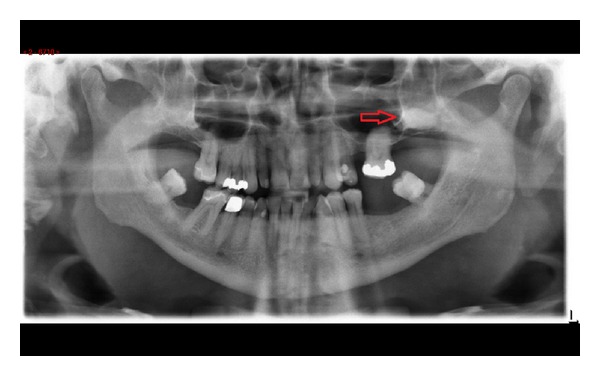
Panoramic radiograph revealing superimposition of left upper third molar and left maxillary sinus (red arrow).
